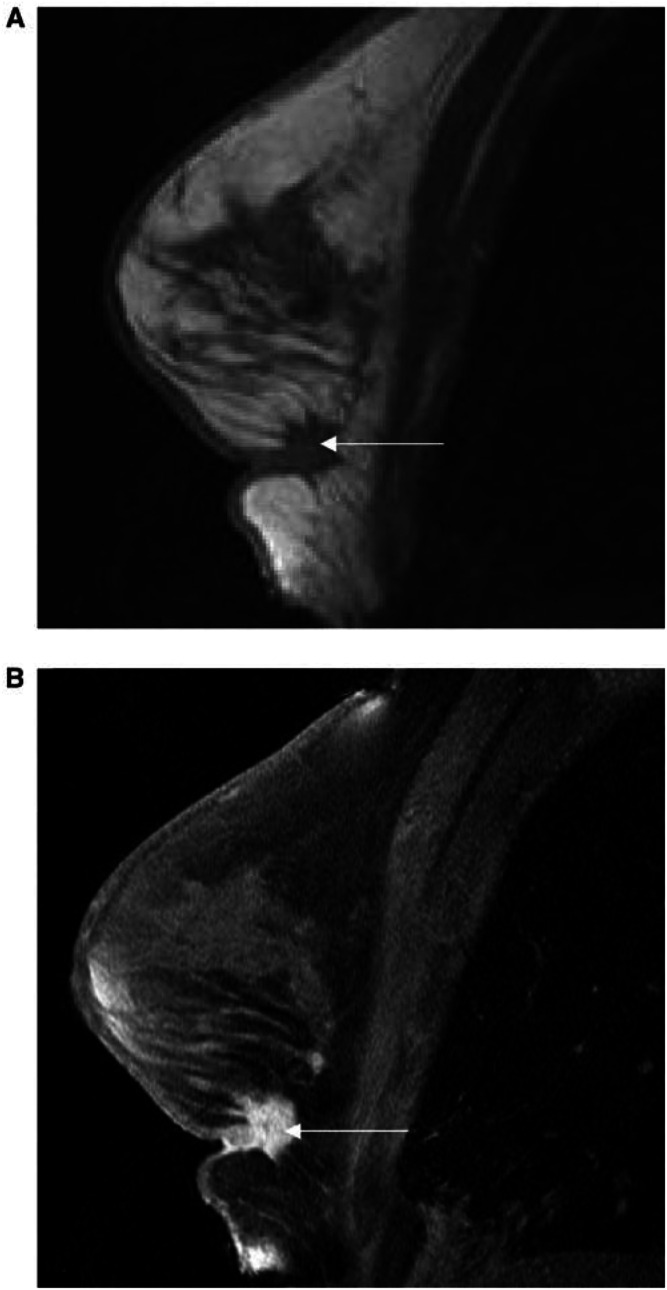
A T1-weighted image of an invasive breast cancer (A) fat-saturated image after gadolinium contrast (B).
